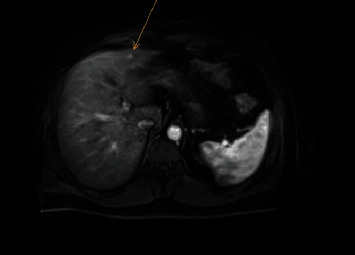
Liver MRI one month earlier demonstrated only one lesion in segment 3 and no lesion in segment 1.
